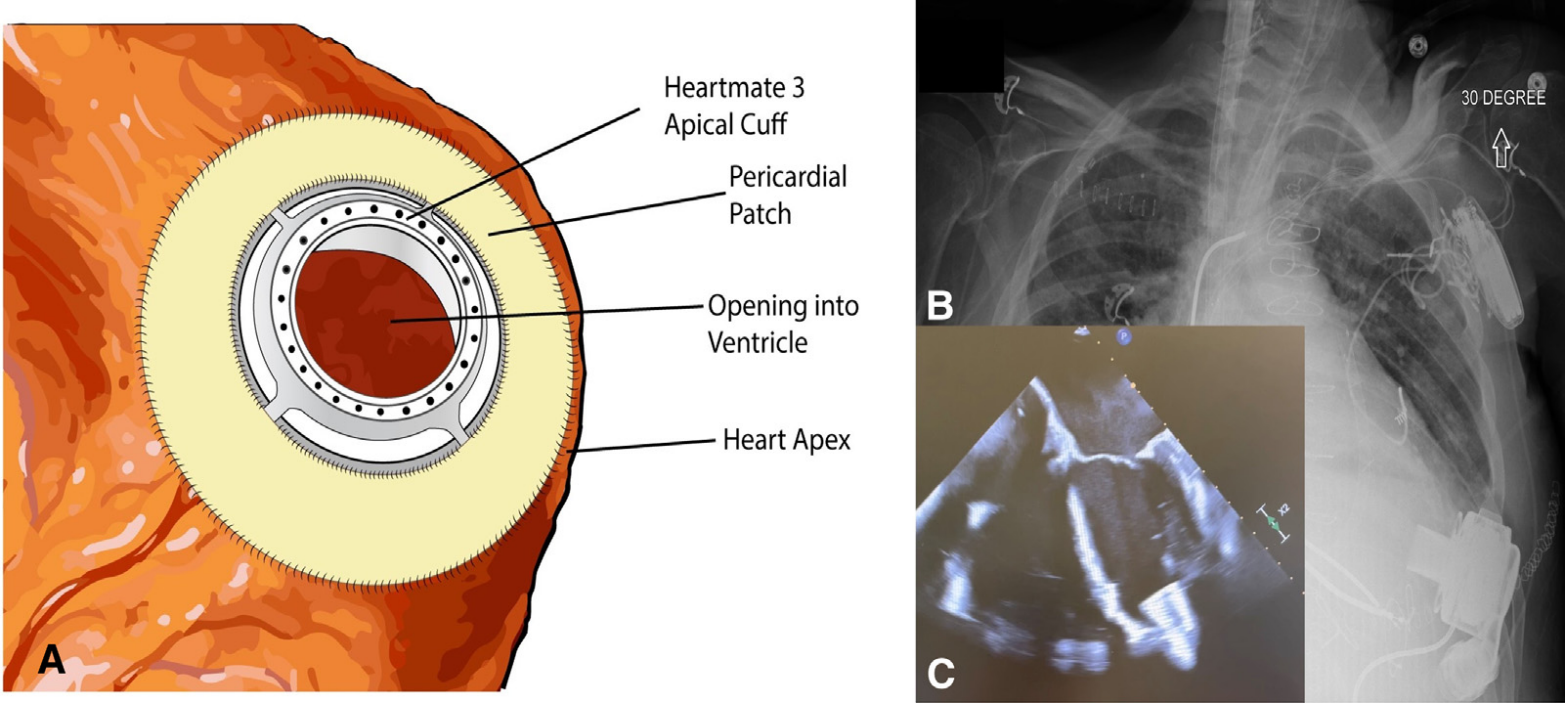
A, Sketch of pericardial patch covering the apical cuff suture lines. Chest radiograph (B) and echocardiogram (C) show good position of the HeartMate 3 inlet in the LV.
